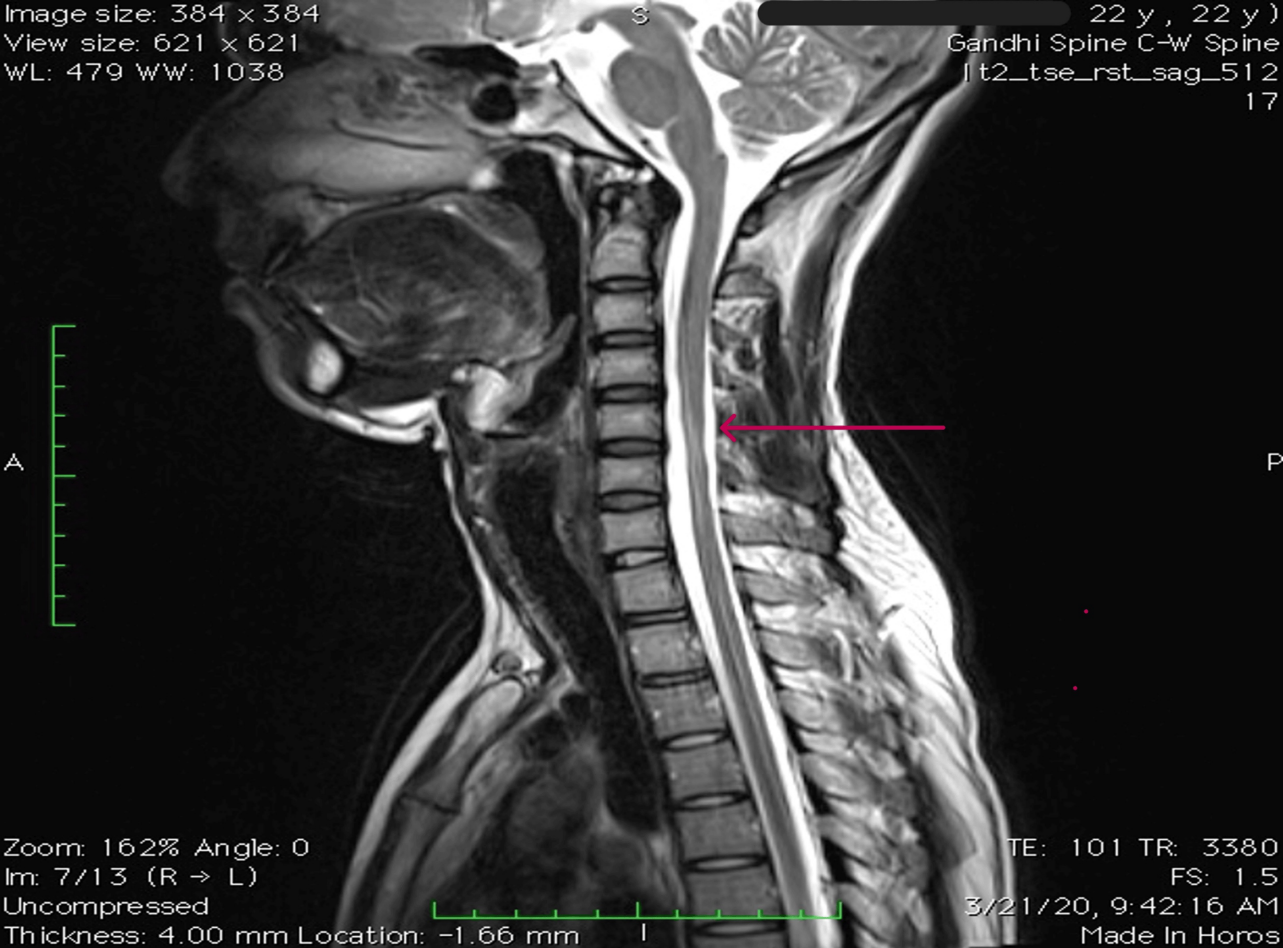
Abnormal T2W sagittal MRI of the neck in a neutral position showing hyperintensity at the site of the maximum forward shift
